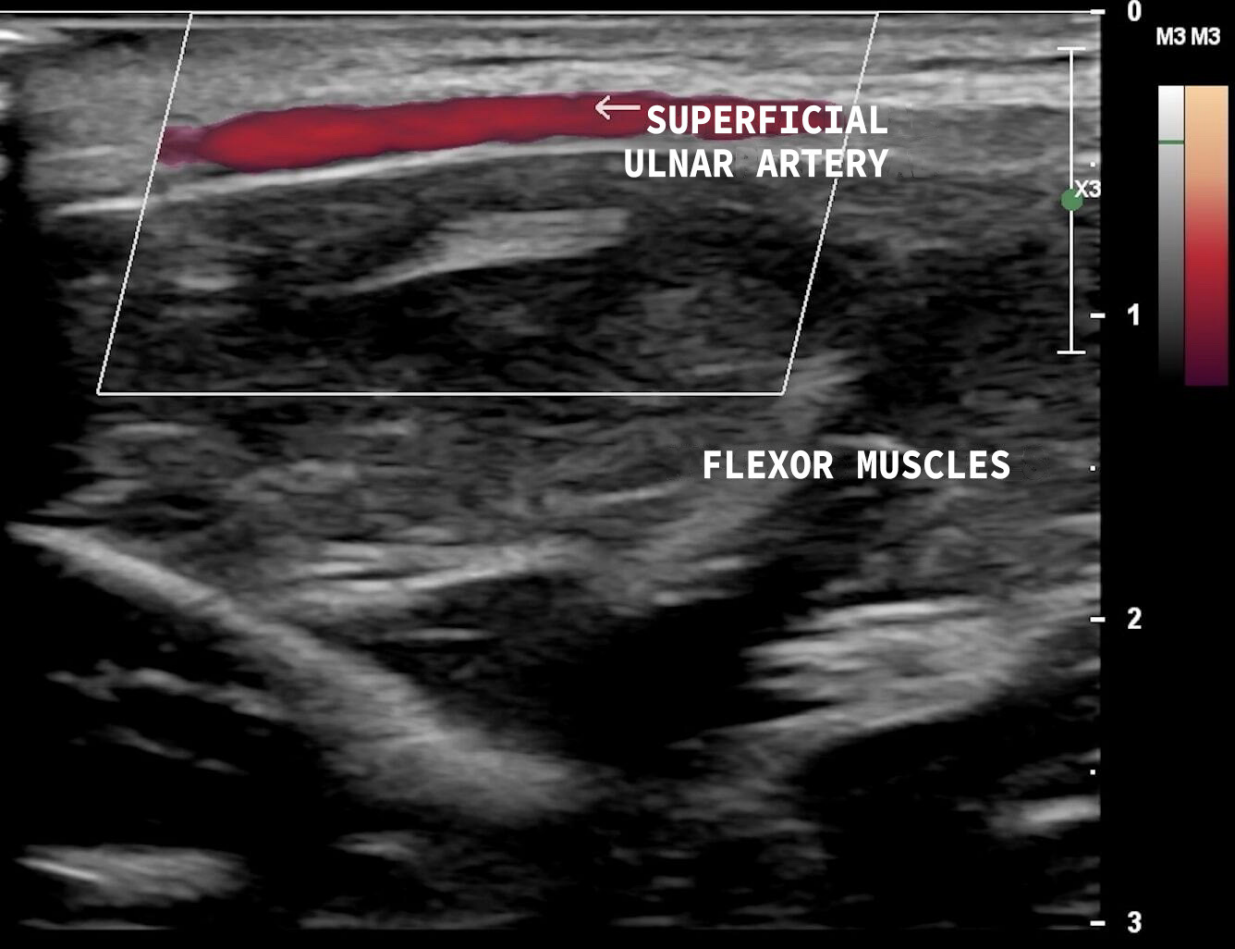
Vascular ultrasound, longitudinal view, with superficial ulnar artery anatomic variant present, coursing above the flexor muscles of the forearm.
